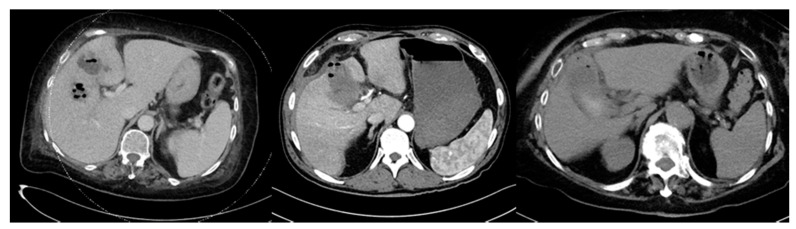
Shows three individual patient’s CT scans with gas bubbles inside the gallbladder as well as one with gas bubbles inside an adjacent liver abscess (far left).
